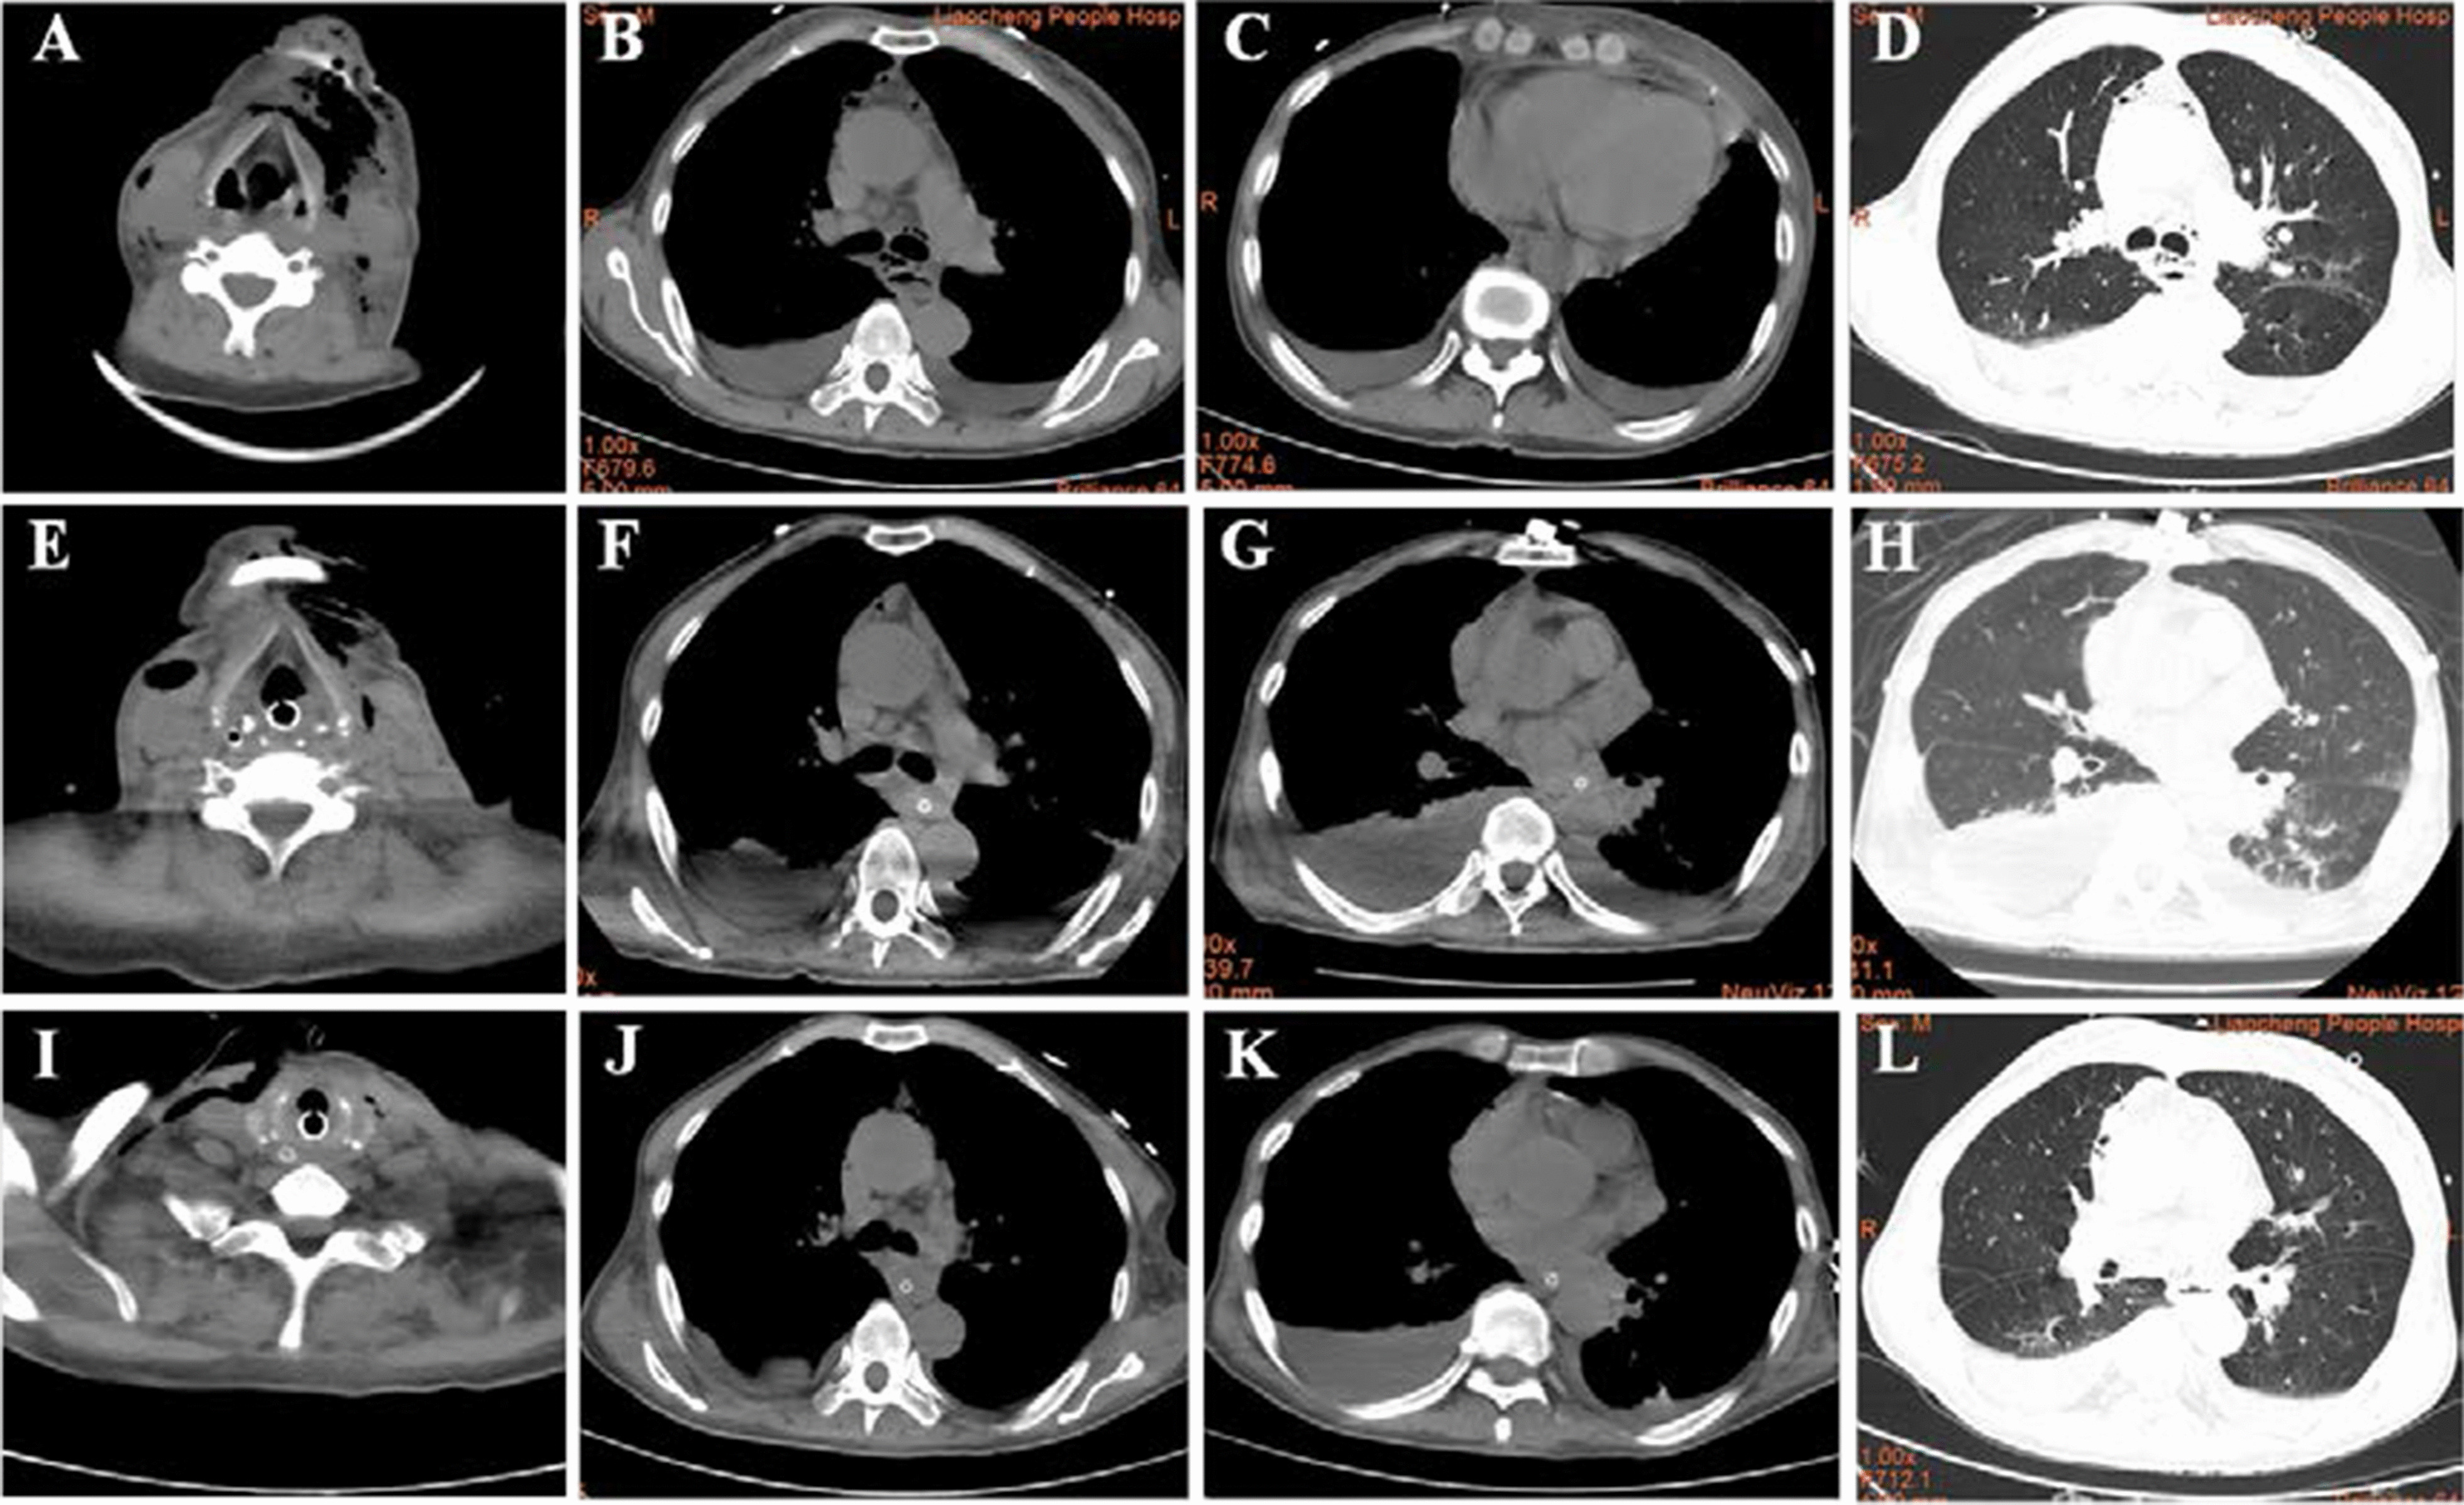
CT images of the patient at
